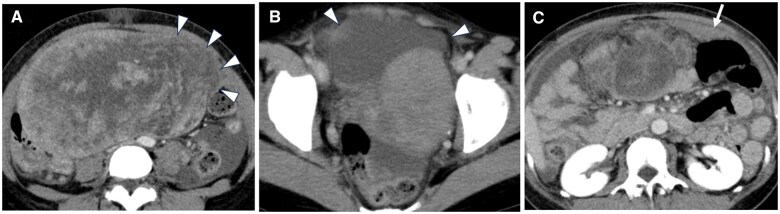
Contrast-enhanced CT at the time of admission due to abdominal pain (1 month after the initial MRI at the previous hospital). (A) The mass has enlarged to 20 cm. The mass is ruptured on the left side, with the contents of the mass protruding at the rupture site (white arrowheads). (B) Pelvic ascites are present, with some areas showing high density, indicative of haemorrhagic ascites. Peritoneal thickening is also noted. (C) At the upper abdominal level, disseminated lesions are observed in the omentum near the site of the mass rupture (white arrow).
